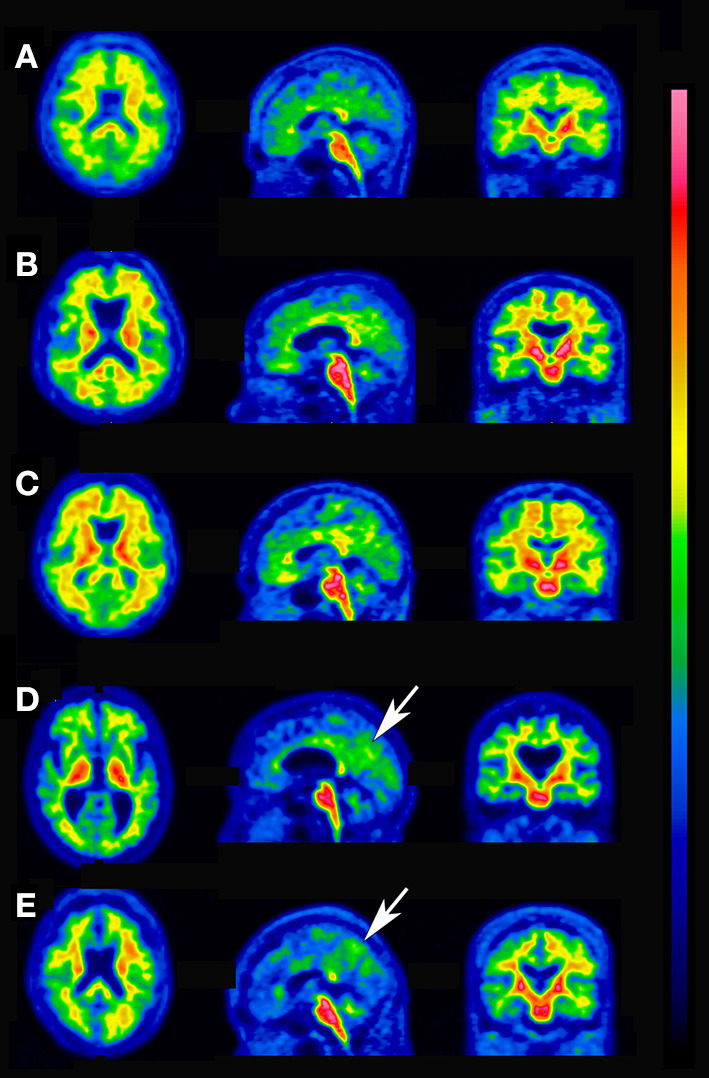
Discordant cases between quantitative assessments and visual interpretation. (A) Visually negative, SUVR 1.22, CL 26, diffuse elevation of cortical activity. (B) Visually negative, SUVR 1.19, CL 23, diffuse elevation of cortical activity. (C) Visually negative, SUVR 1.16, CL 19, diffuse elevation of cortical activity. (D) Visually positive, SUVR 1.10, CL 12, focal uptake is present in the precuneus (arrow). (E) Visually positive, SUVR 1.00, CL 1, focal uptake is present in the precuneus (arrow).
